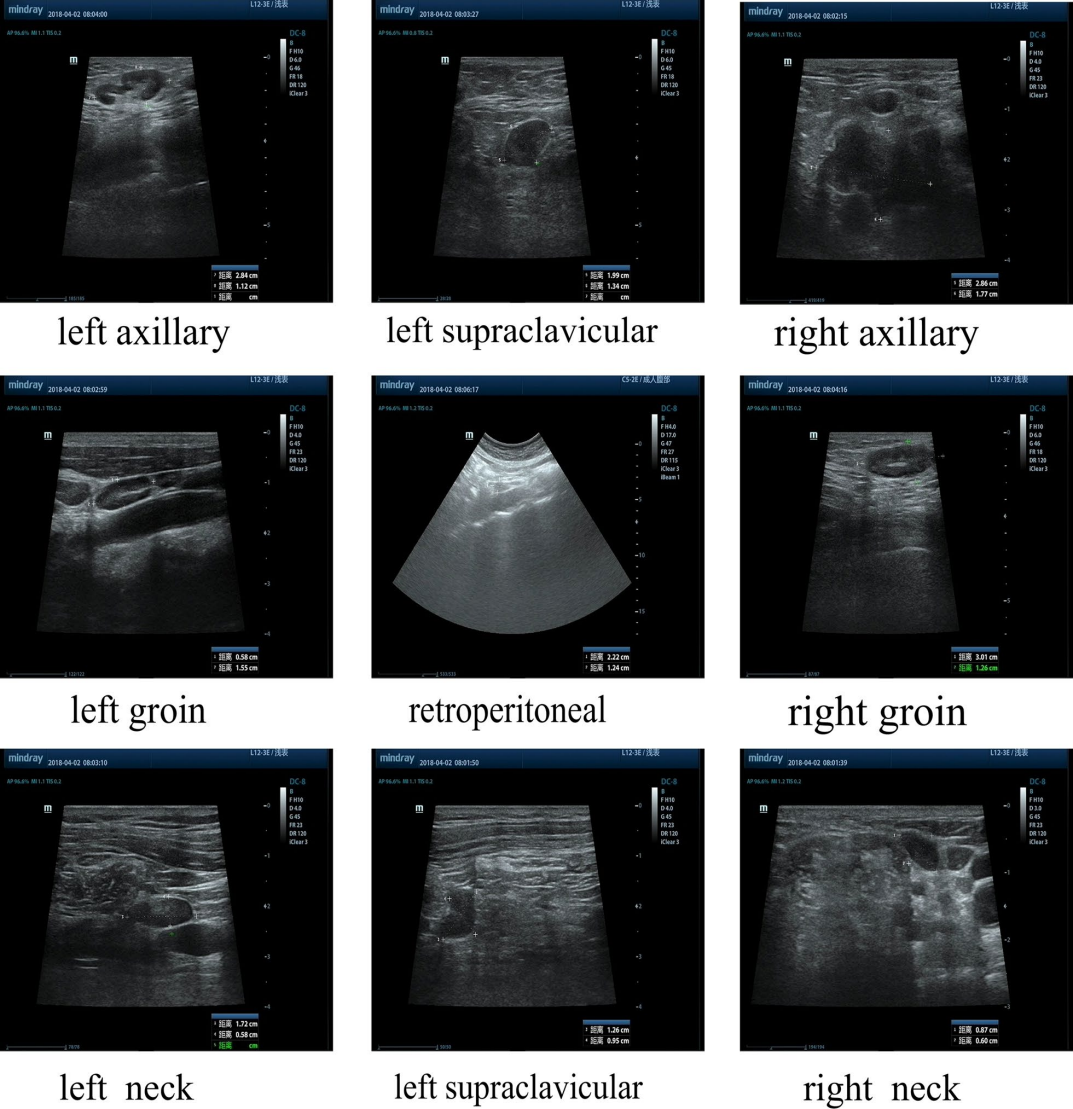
Ultrasonography showing enlarged lymph nodes bilaterally
